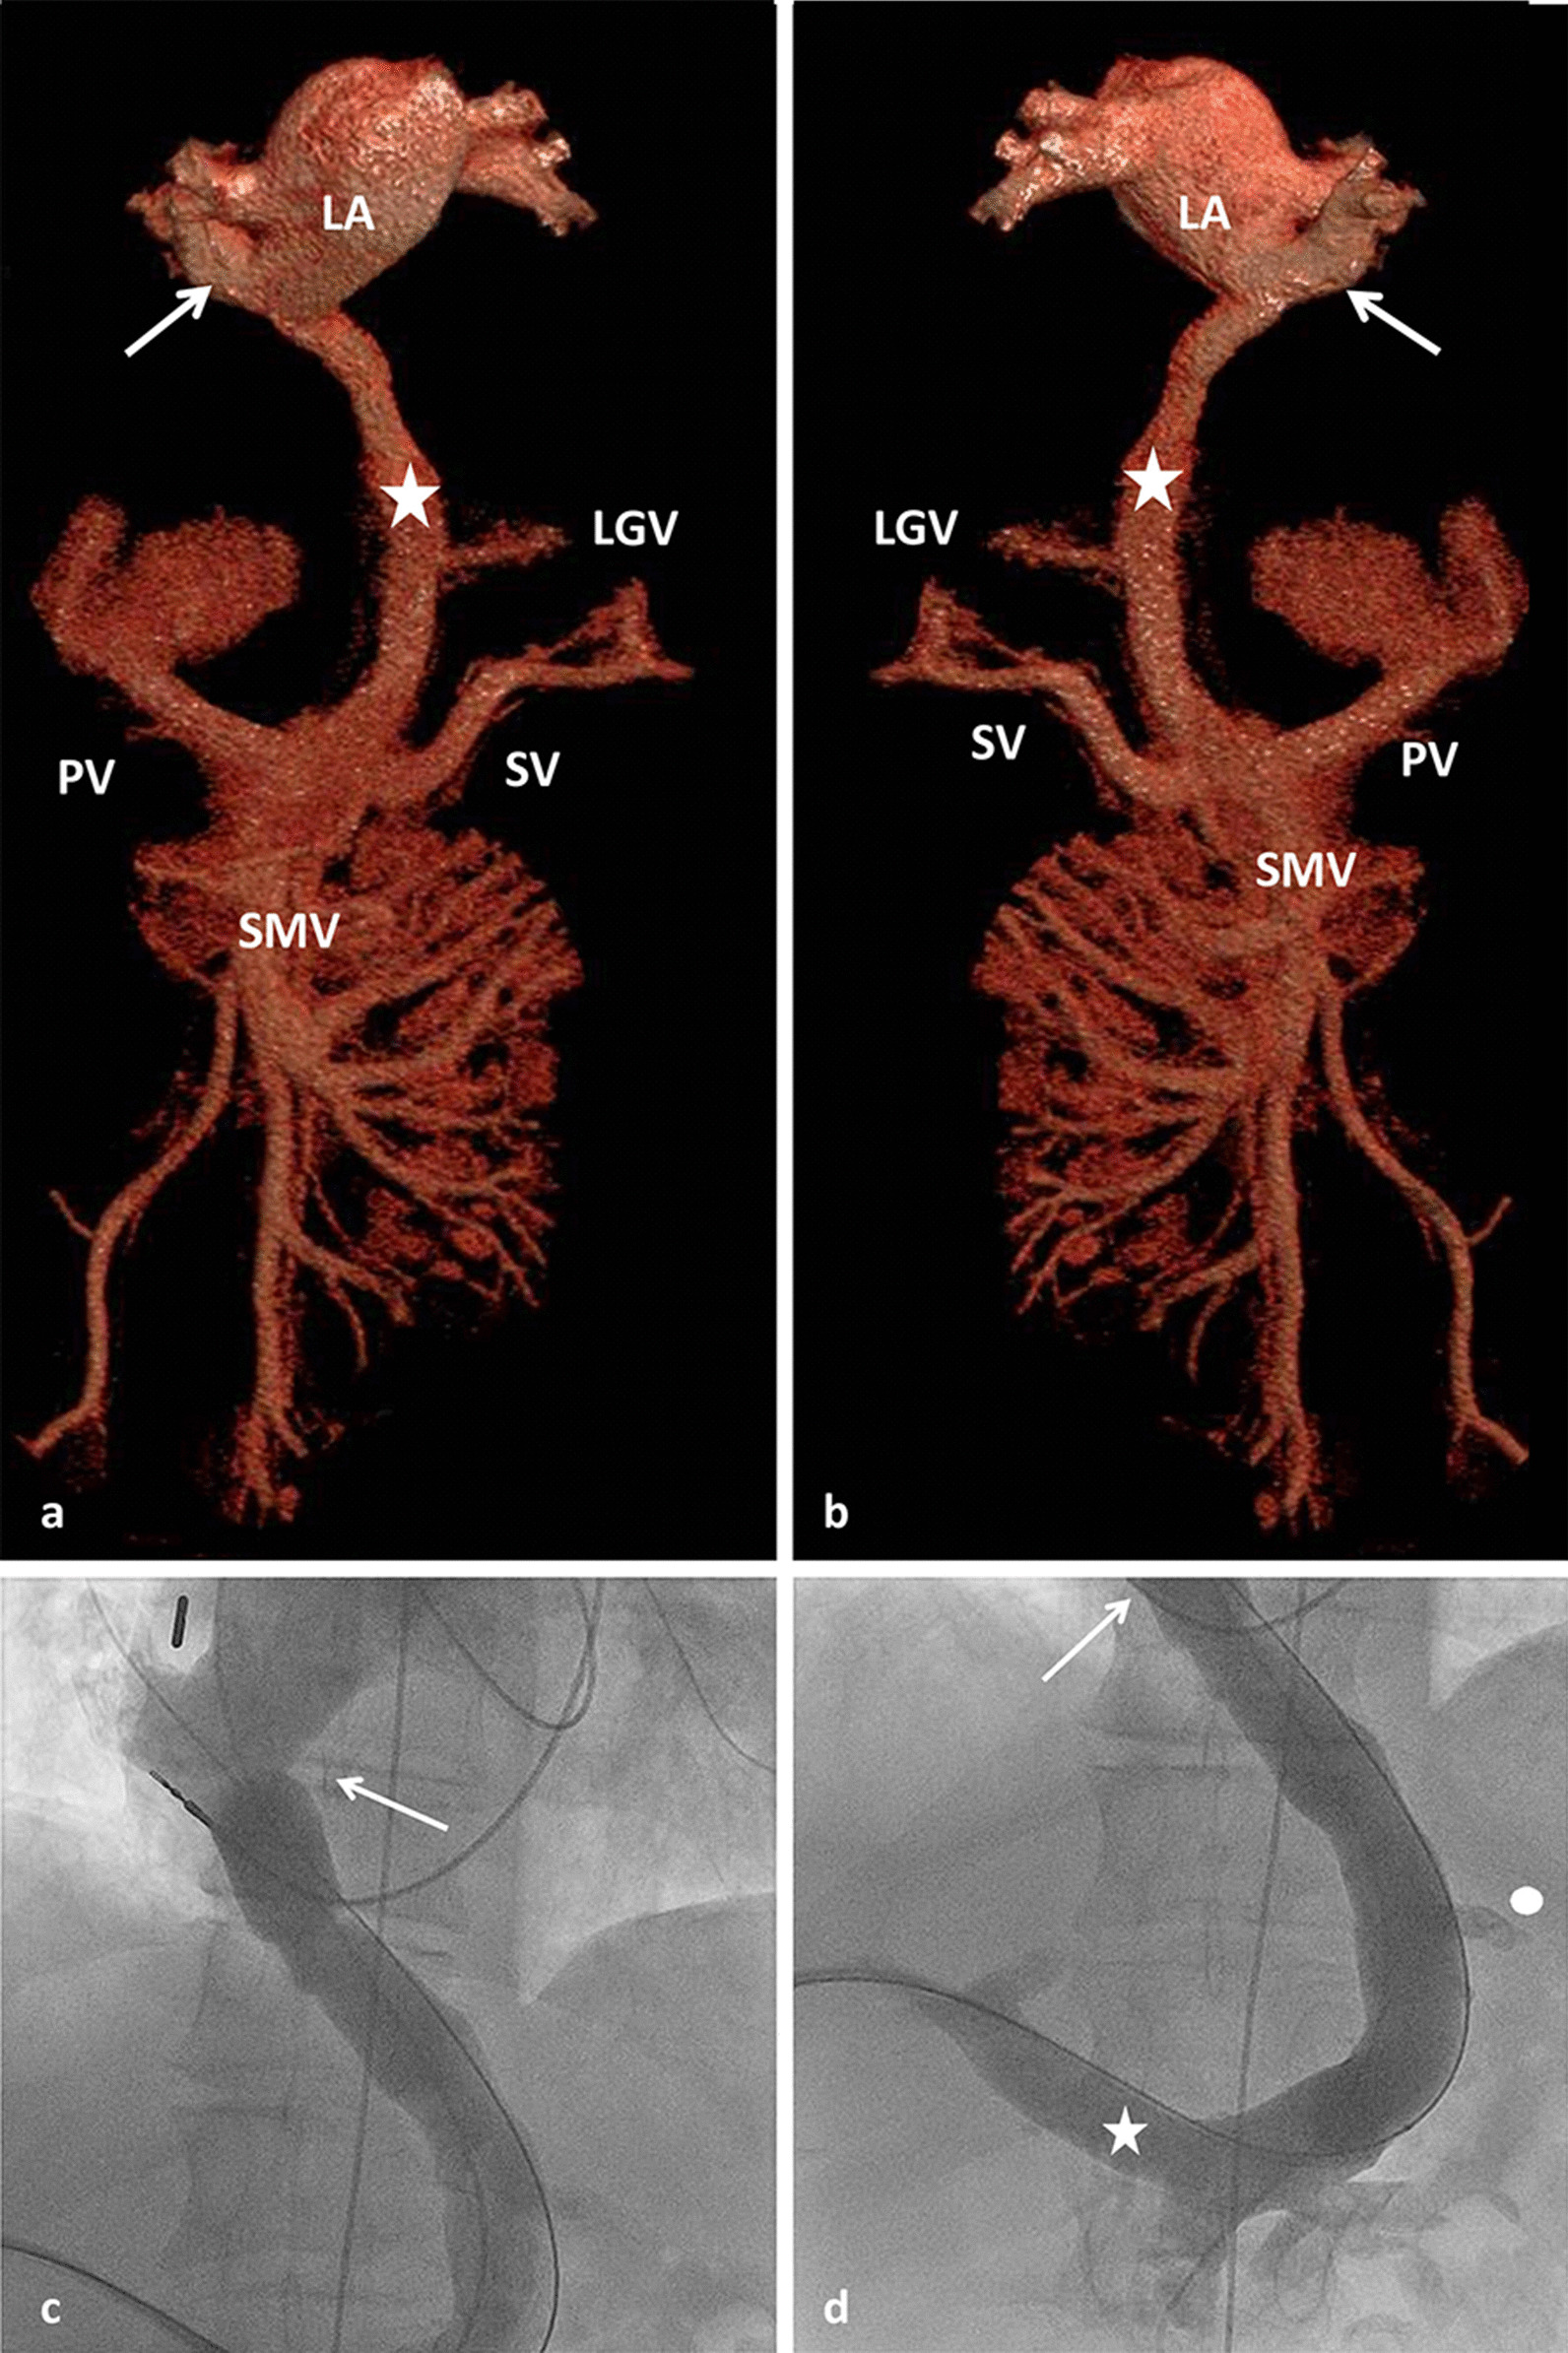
a CT reconstruction (ap-view) showing the shunt vein (star) connecting the portal vein (PV) to the right lower pulmonary vein (arrow). SV: splenic vein, SMV: superior mesenteric vein, LGV: left gastric vein, LA: left atrium. b CT reconstruction (pa-view) showing the shunt vein (star) connecting the portal vein (PV) to the right lower pulmonary vein (arrow). SV: splenic vein, SMV: superior mesenteric vein; LGV: left gastric vein, LA: left atrium. c Angiography confirming the anastomosis (arrow) of the shunt vein to the right lower pulmonary vein, which opens into the left atrium. d Transhepatic access of the portopulmonary connection. Angiography showing the shunt vein extending from the portal vein (star) toward the inferior pulmonary vein (arrow); left gastric vein (circle)
